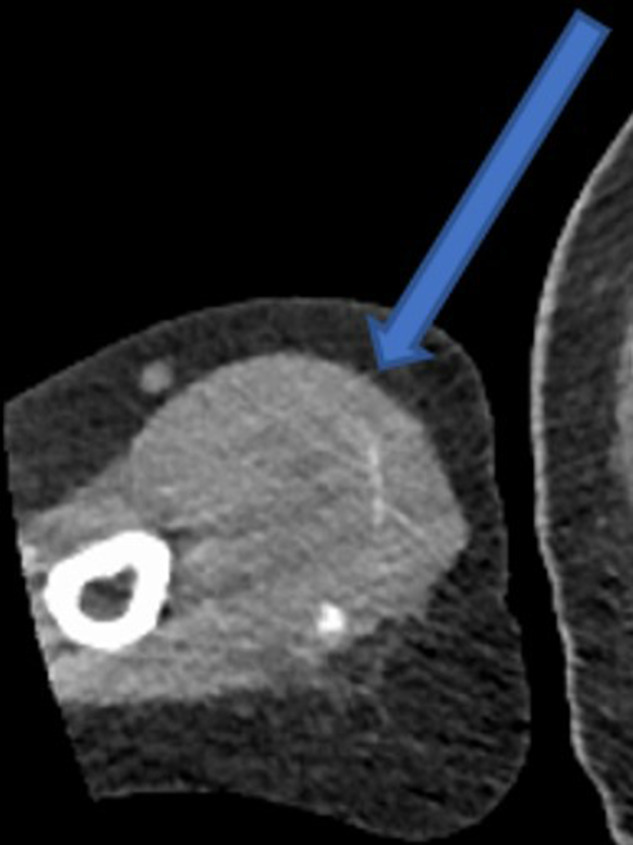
Axial CT image showing muscle lesion in the right arm (arrow).
